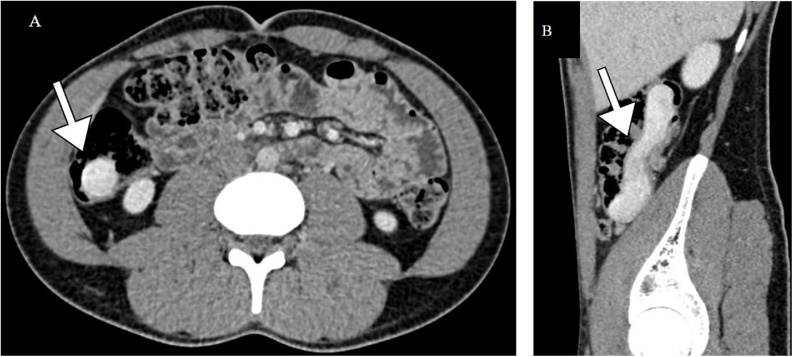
CT examination. A CT examination showed an encapsulated formation with high attenuation in the inner layer of the bowel wall. The lesion is marked by an arrow in axial image (A) and sagittal image (B).
